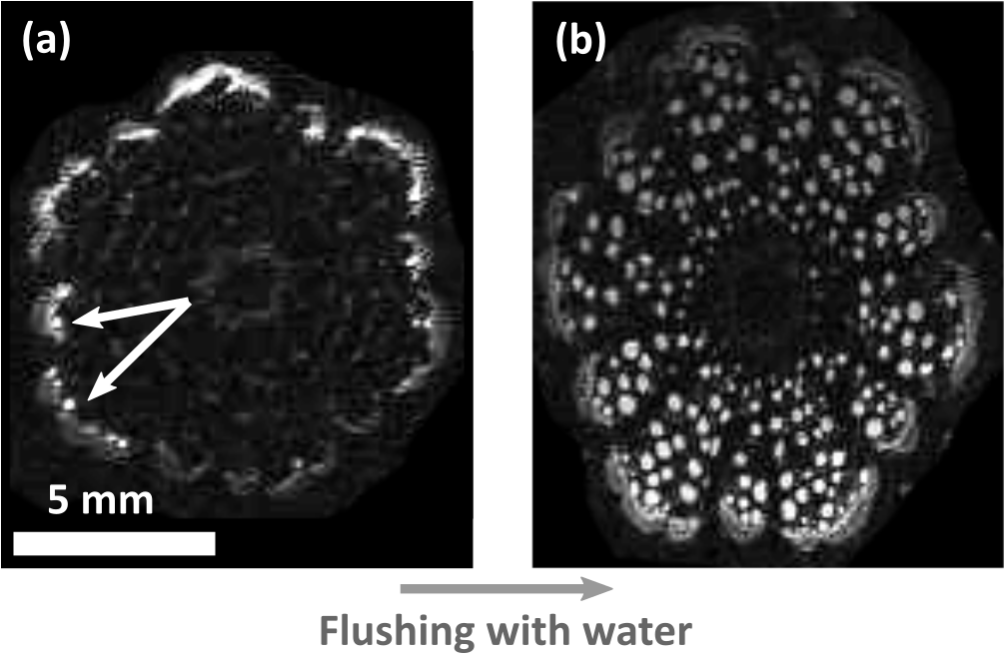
Magnetic resonance images of *Clematis vitalba* stem. (A) Living plant. The functional conductive xylem area is restricted to the outermost growth ring (white conduits, indicated by white arrows). Only a few active vessels were detected. (B) Flushed cross section. Conduits of all growth rings are water-filled after flushing. The images were equally contrasted by image analysis to highlight the functional conduits.
